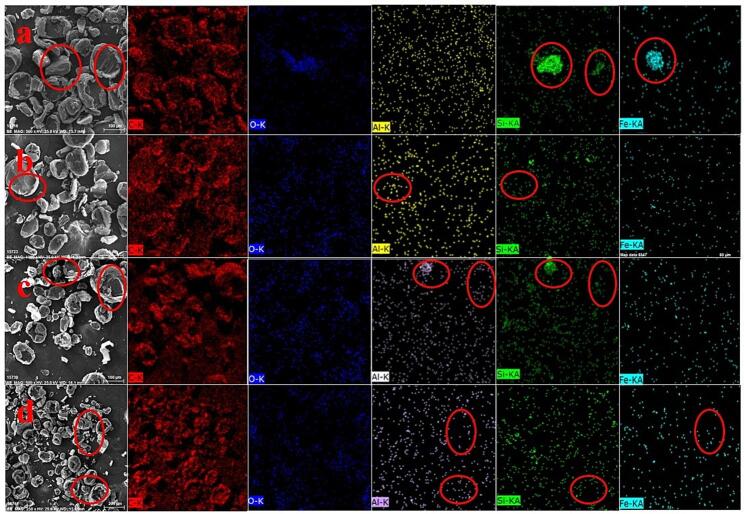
SEM-EDS images of graphite flotation concentrate pre-treated by different methods. (a: graphite ore; b: horn-type ultrasound, intensity of 100%, 1 min; c: bath-type ultrasound, intensity of 100 %, 4 min; d: Combination of two types of ultrasounds with the horn-type ultrasound intensity of 100% and bath-type ultrasound intensity of 60%, 1 min).
